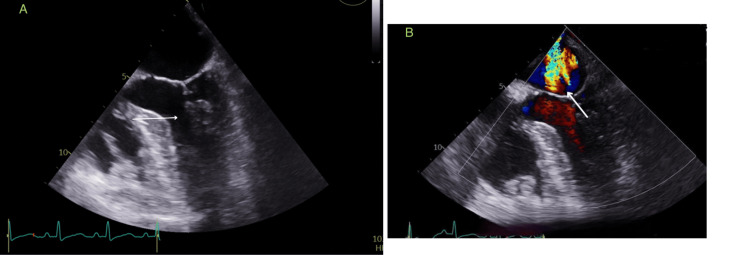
Transesophageal echocardiogram Image A: The arrow showed Posterior Mitral valve chordae originating from single papillary muscle Image B: color doppler, arrow showed significant Mitral Regurgitation
